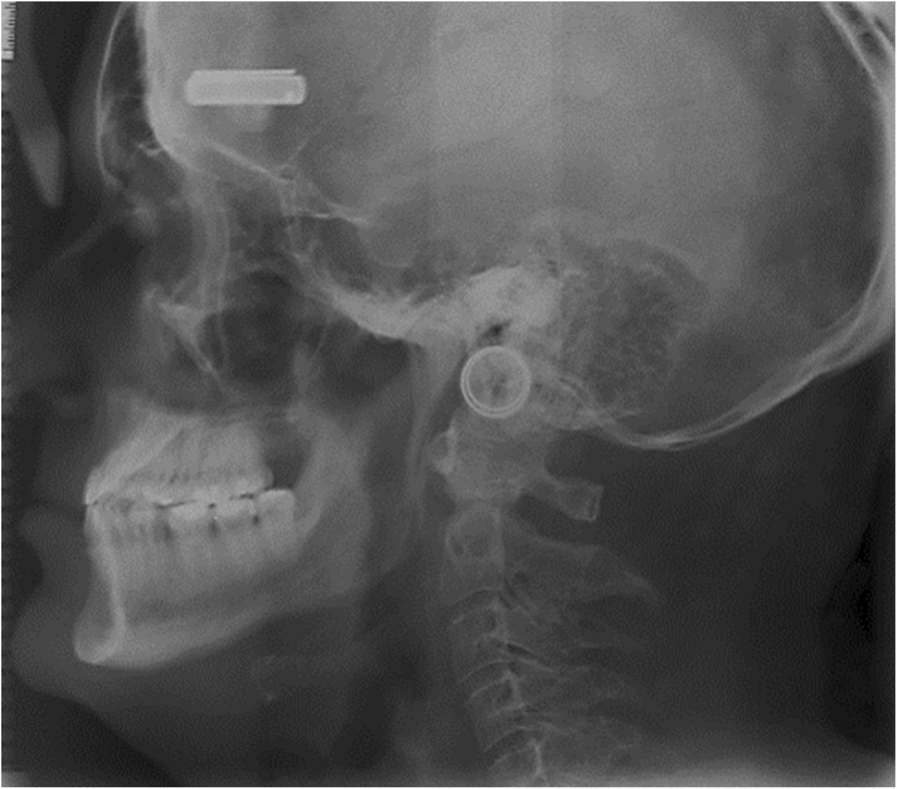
Lateral skull radiograph of a 53-year-old man with NF1 shows marked maxillary retrognatia
